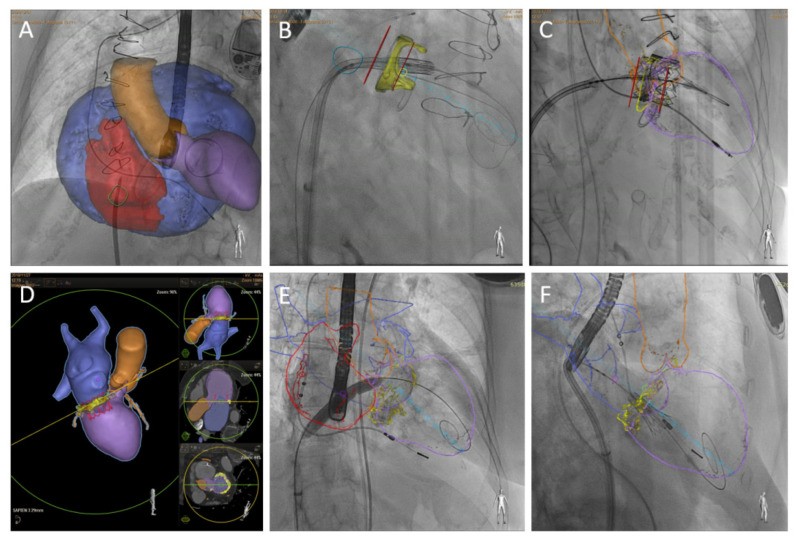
CT-fluoroscopy fusion imaging. The superior row shows a TMVR valve-in-valve procedure in a patient with extreme left atrium enlargement and a modified projection required for transeptal puncture (A). Markers (red lines) may be over-imposed on fluoroscopy imaging to guide depth deployment (B,C). Inferior row, TMVR valve-in-MAC CT preprocedural planning (D), interatrial septal balloon dilatation (E), and initial phase of THV deployment with coaxial projection to mitral annulus (F). Reproduced from ref. [4] published by MDPI, Basel, Switzerland, under the Creative Commons Attribution License (CC BY) [4].
